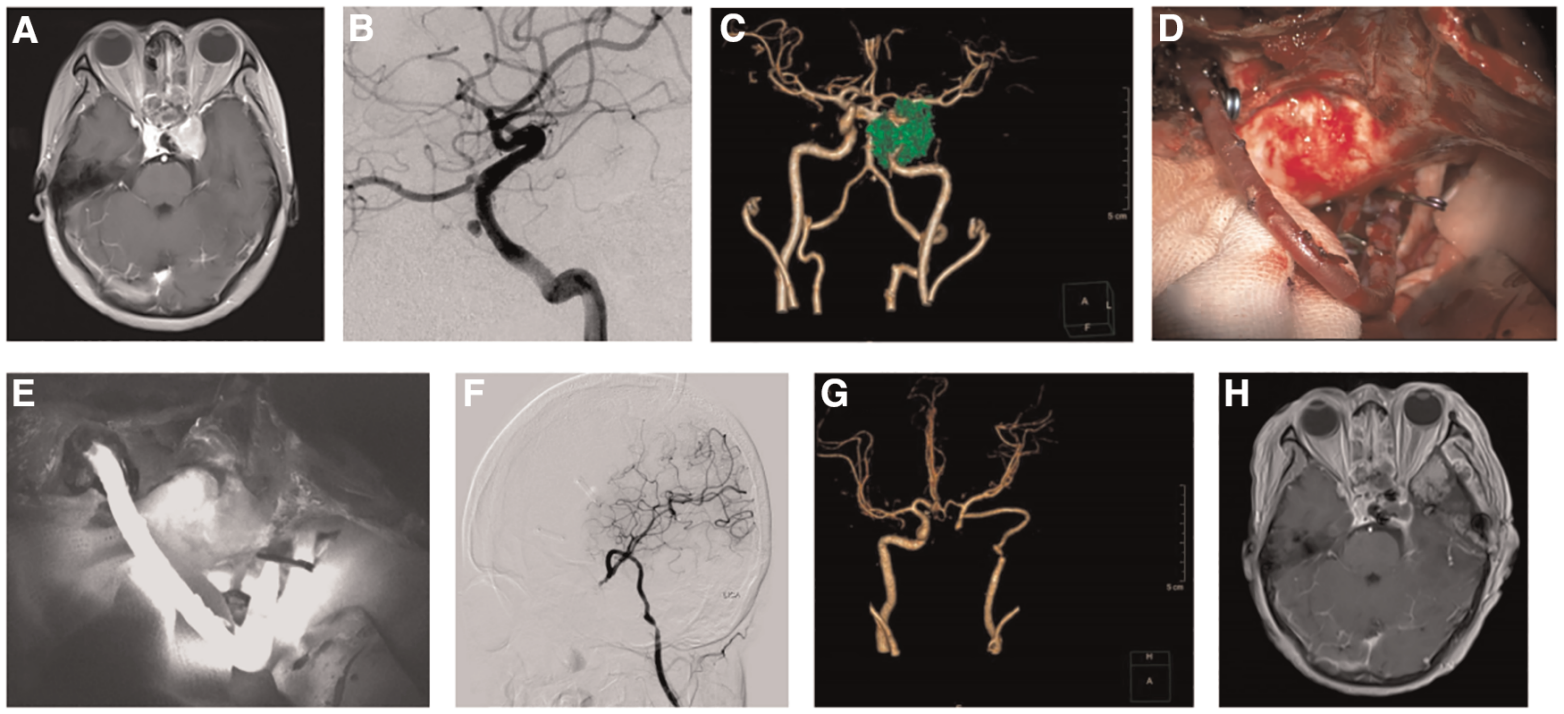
Images of a patient before and after Fukushima bypass type I. (A) Enhanced magnetic resonance imaging (MRI) showing a pituitary adenoma in the sellar region involving the cavernous sinus and encasing the left internal carotid artery. (B) Digital subtraction angiography (DSA) showed pseudoaneurysm of the internal carotid artery. (C) Computed tomography angiogram (CTA) showing tumor encasing the left internal carotid artery and aneurysm. (D). Intraoperative image of Fukushima bypass. (E) Intraoperative fluorescein angiography showing patent bypass blood flow. (F) Postoperative reexamination of MRI showing subtotal resection of the tumor. (G) Postoperative CTA showing patent blood flow in radial artery graft. (H) Postoperative DSA showing patent blood flow after vascular anastomosis.
